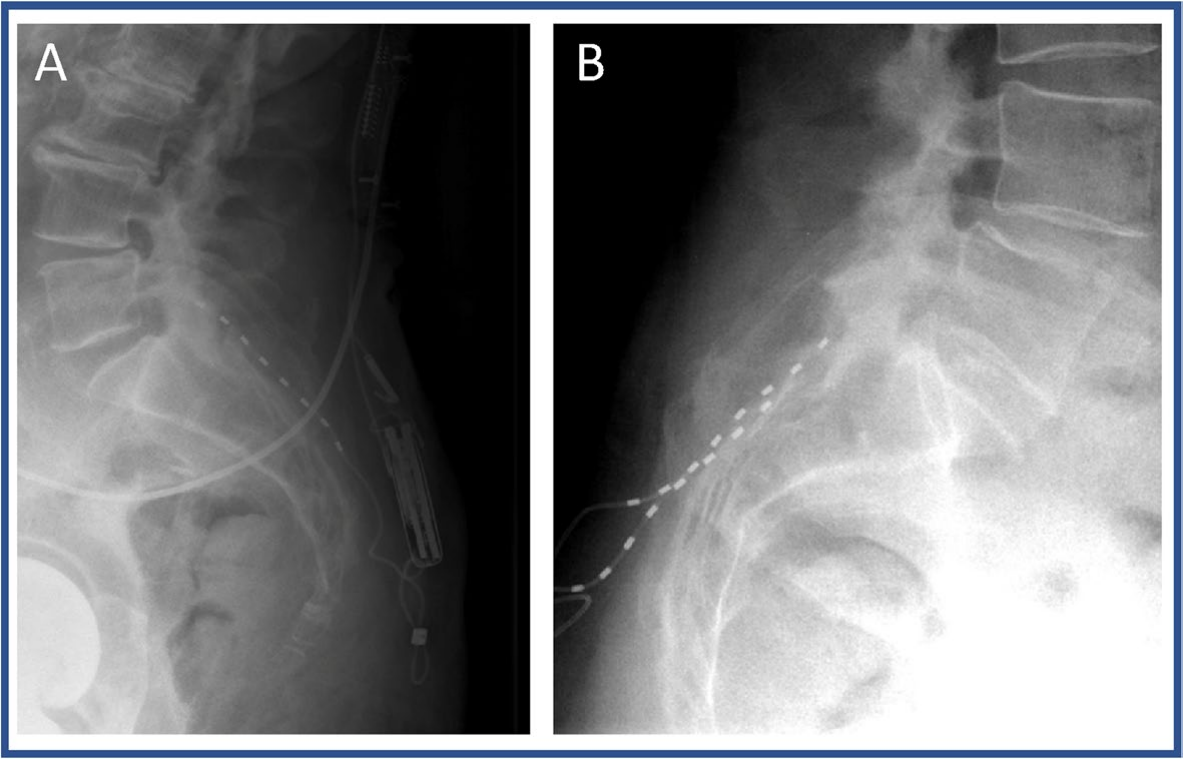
Postoperative sagittal X-rays of two different patients with placement of the leads close to the sacral surface. A Patient with unilateral lead. B Patient with bilateral leads
